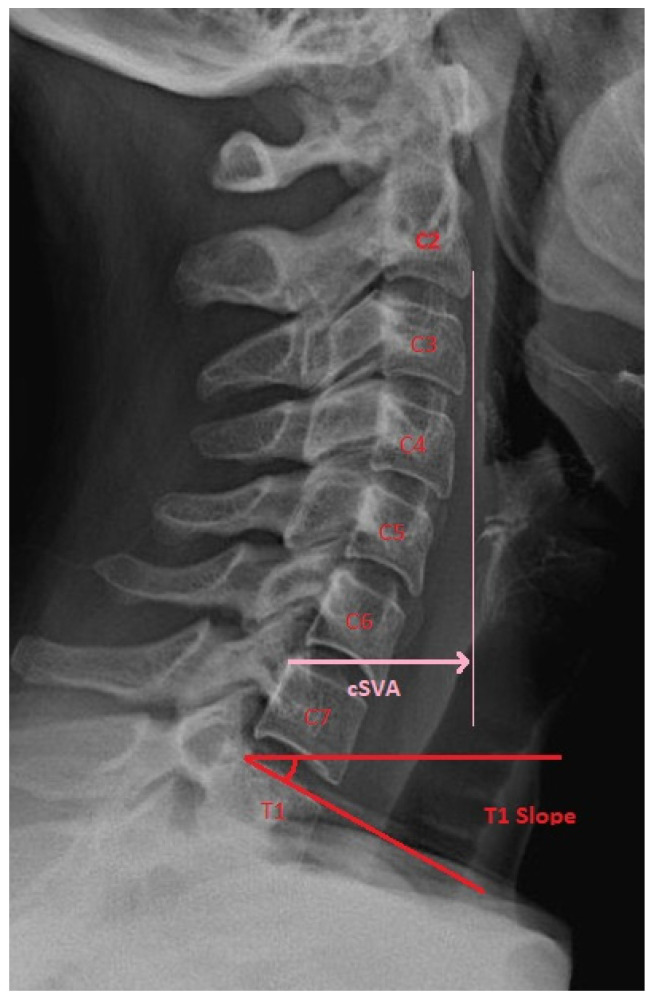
Lateral cervical spine radiograph demonstrating cervical sagittal vertical axis (cSVA) and T1 slope.
